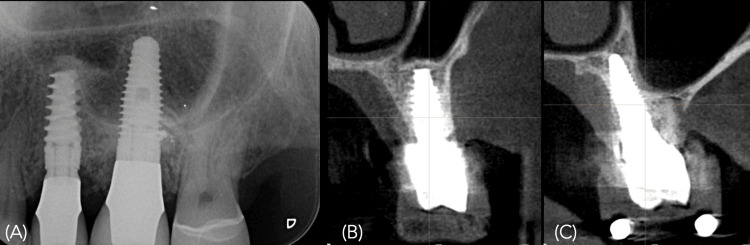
Radiographic images A) Periapical radiograph immediately after the delivery of the ceramic crowns at 25 and 26. Note that the palatal implant placement may create a radiographic impression of a lack of surrounding bone; B) Cone-beam computed tomography (CBCT) scan of the implant at 25; C) CBCT scan of the implant at 26 confirming that the final result corresponded exactly to the virtual plan, with circumferential bone surrounding the implant.
